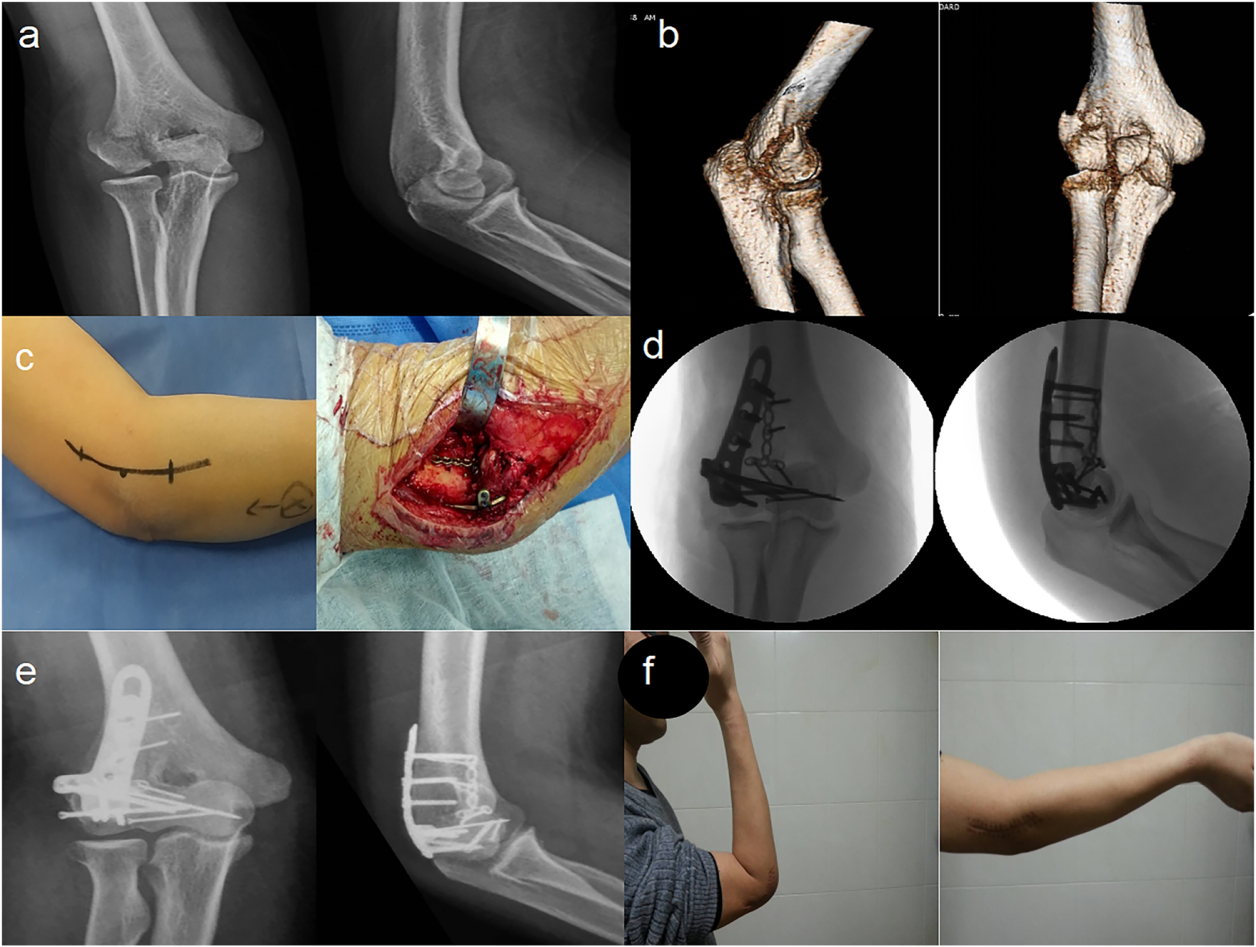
Dubberley type 3B fracture: a A 37-year-old male had a coronal shear fracture of distal humerus (Dubberley 3B) with preoperative X-ray images of distal humerus. b Preoperative CT images showed seperated fragments of capitellum and trochlea and comminution of posterior condylar. c Extended lateral incision was applied. f Intraoperative image of a micro-T-shape plate and a locking compression plate was showed. d Intraoperative C-arm images were evaluated. e postoperative X-ray images showed anatomic reduction and rigid fixation using all surgical techniques. f The patient was satisfied with the final outcome at his 1-year visit, and there was no significant influnce in his noraml activity.
